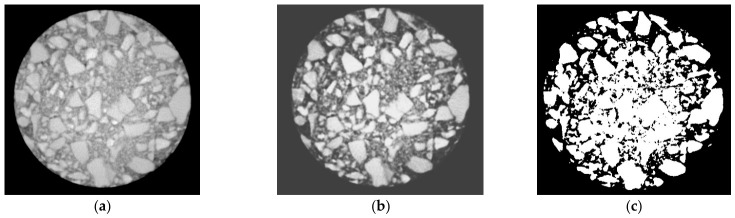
CT scanning cross-section image processing of SFPM (Sacle bar: 25 mm). (a) Grayscale image. (b) Image after equalization. (c) Multi-threshold segmentation.
